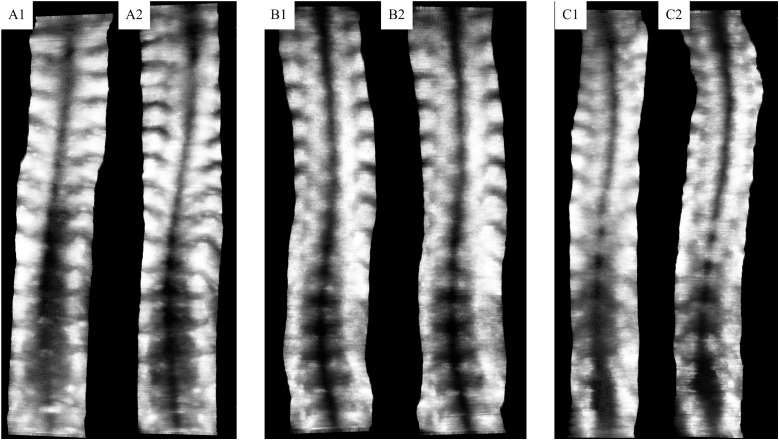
Spinal ultrasound images of subjects (A&B&C) with different BMIs across two scans (1&2). A: underweight (BMI 13.7 kg/m^2^); B: Normal-weight (BMI 17.4 kg/m^2^); C: Overweight (BMI 24.1 kg/m^2^).
